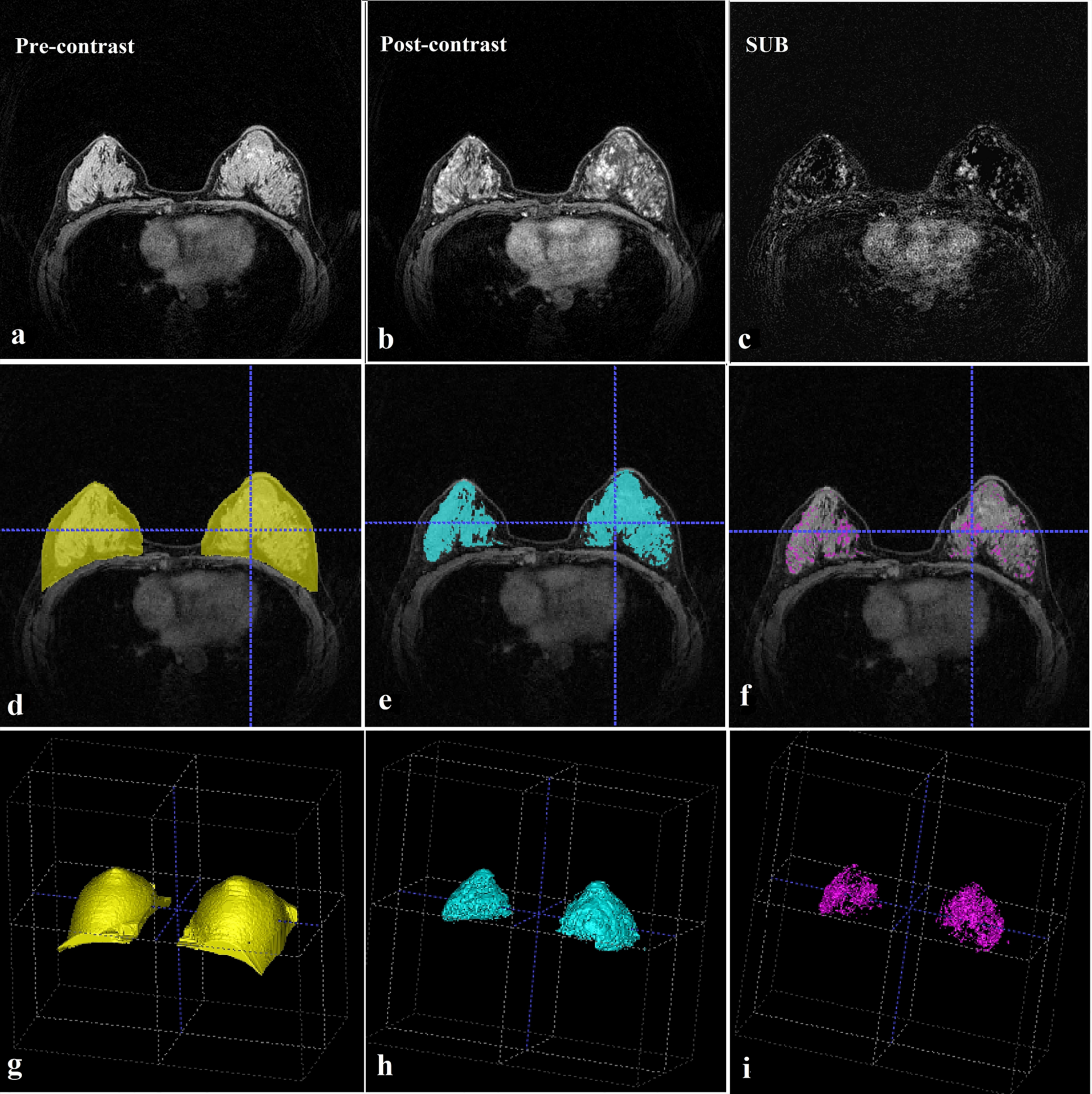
MRI evaluation. (A) Pre-contrast breast MRI (T1-weighted fat-suppressed sequence). (B) This post-contrast (early) image corresponds to the T1-weighted image shown in (A). (C) Subtraction image from (A, B). (D) Whole breast segmentation (yellow). (E) Fibroglandular tissue segmentation (blue). (F) Enhanced fibroglandular tissue segmentation (purple). (G) Whole breast segmentation (3D). (H) Fibroglandular tissue segmentation (3D). (I) Enhanced fibroglandular tissue segmentation (3D). MRI = magnetic resonance imaging, 3D = three-dimensional.
